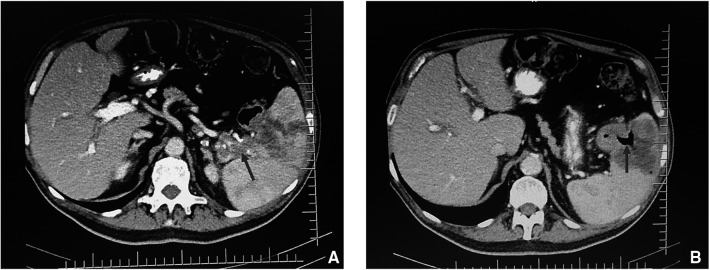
CT scan of the thorax and abdomen showing (A) suspicious fistulous tract between colon and spleen (arrow) and (B) air in the spleen (arrow).
